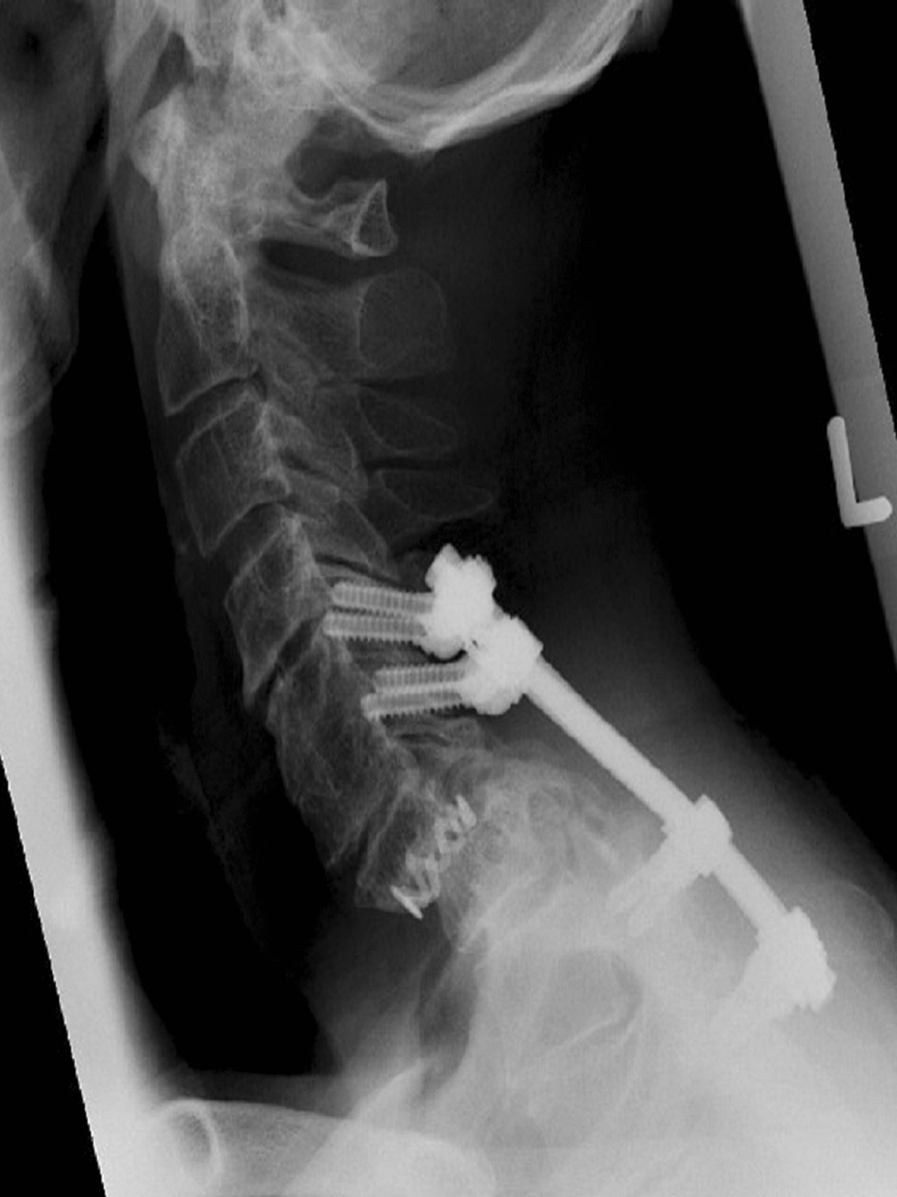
Lateral radiograph of the cervical spine shows the treatment of the spondylolisthesis at C7-T1.
